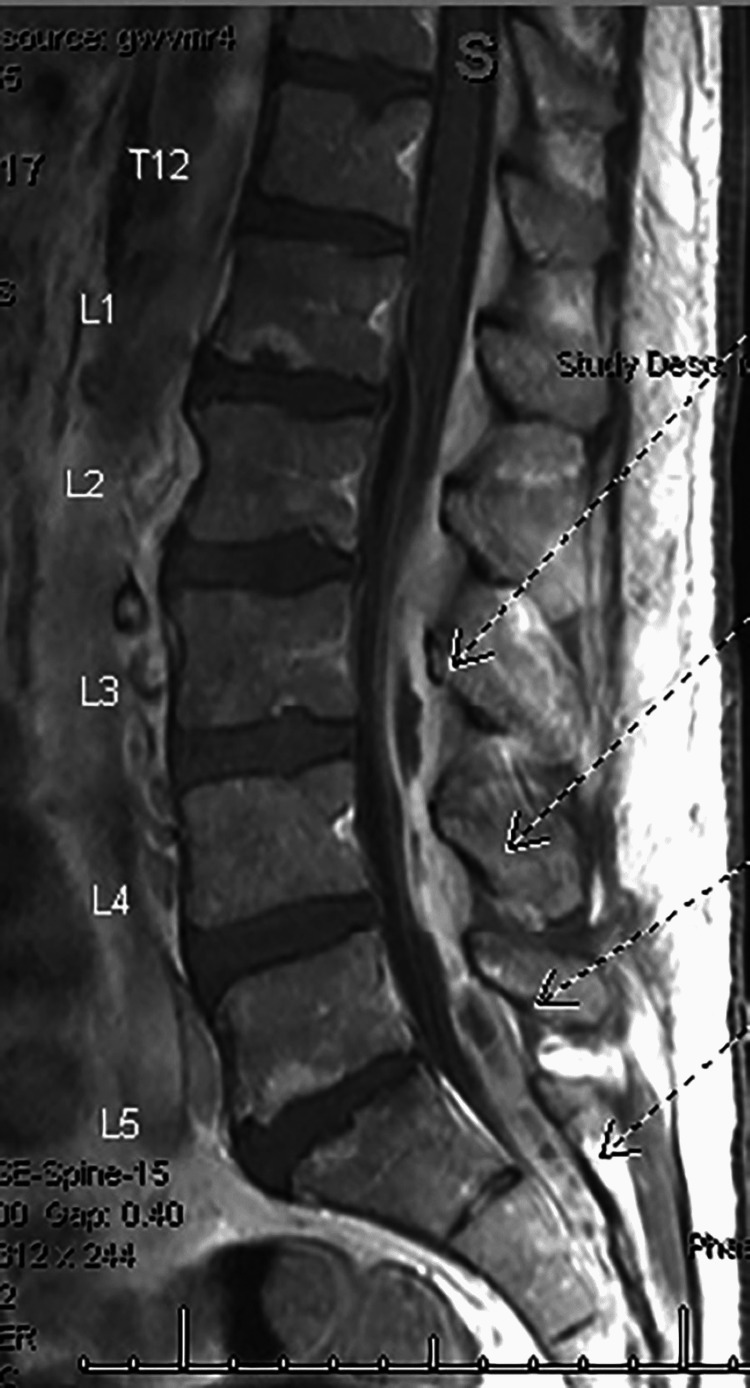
MRI (SAG T1 + contrast sequence) showing a long segment of epidural enhancement compatible with epidural phlegmon/abscess, extending T12 through the imaged sacral levels, which contributes to a varying degree of the spinal canal and neural foraminal narrowing SAG: Sagittal.
